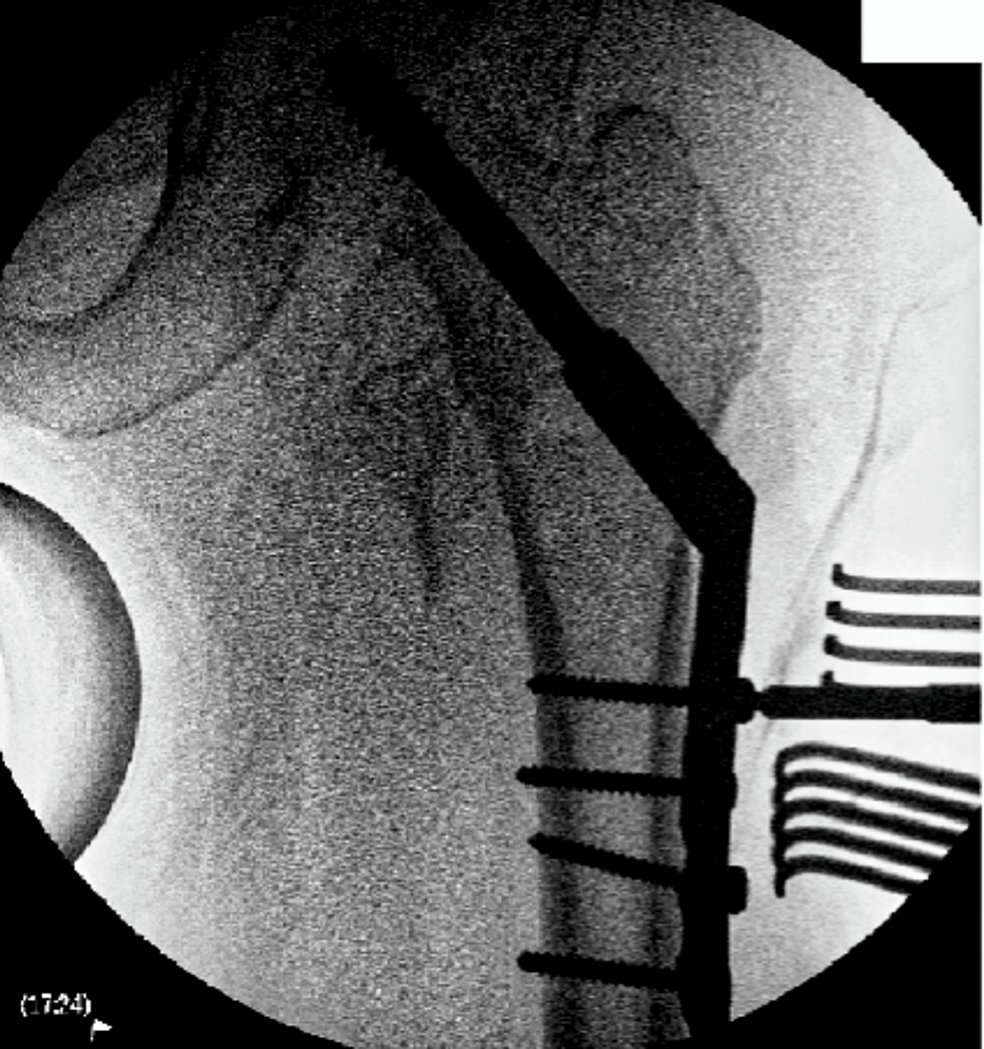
Intraoperative final X-ray of dynamic hip screw fixation.
